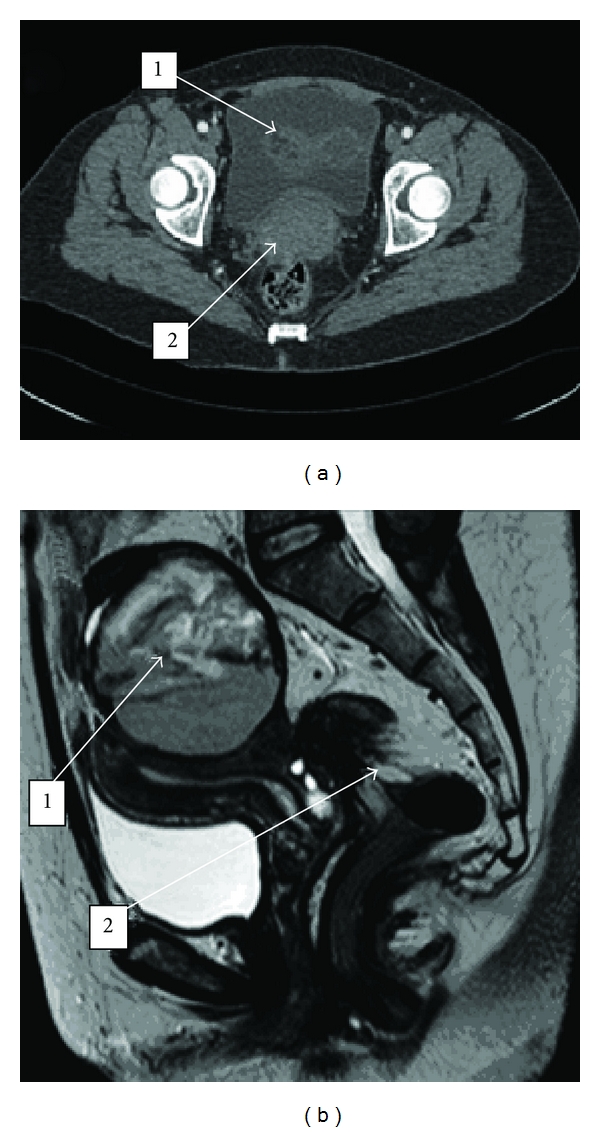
(a) An axial CT angiogram of the pelvis performed 3 months after delivery. (1) indicates a pelvic mass. (2) demonstrates a bulky cervix with a surrounding ill-defined outline where an underlying cervical malignancy could not be ruled out at this point. (b) T2 sagittal MRI scan taken 4 months after delivery. (1) highlights pelvic endometriosis with a left endometrioma sitting over the uterine fundus. (2) demonstrates tethering of the plaque to the ventral rectosigmoid junction indicating the severity of the endometriosis.
